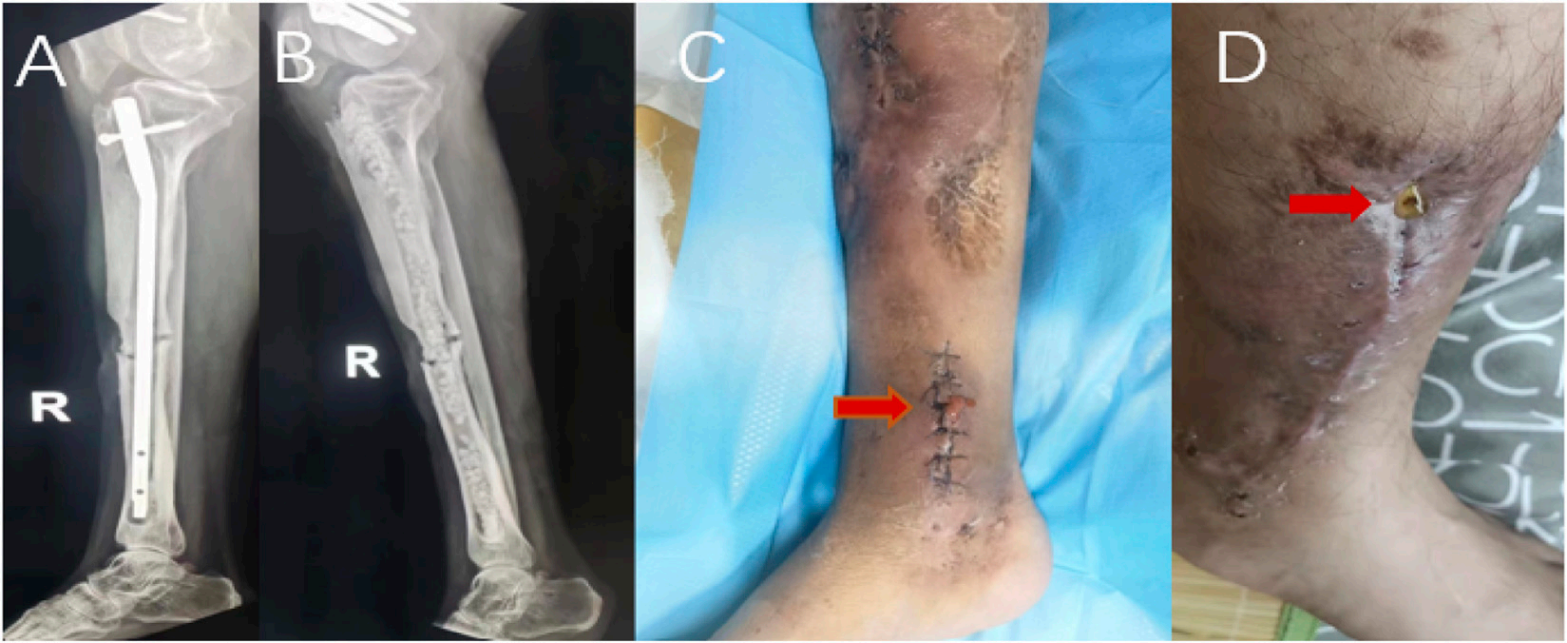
Male patient, 51 years old, X-ray before operation (A), X-ray 1 day after the operation (B), serous exudation appearance of incision on the 6th day after antibiotic-loaded calcium sulfate implantation (C).Another male patient, antibiotic-loaded calcium sulfate implantation 19 days after incision serous exudation appearance (D).
